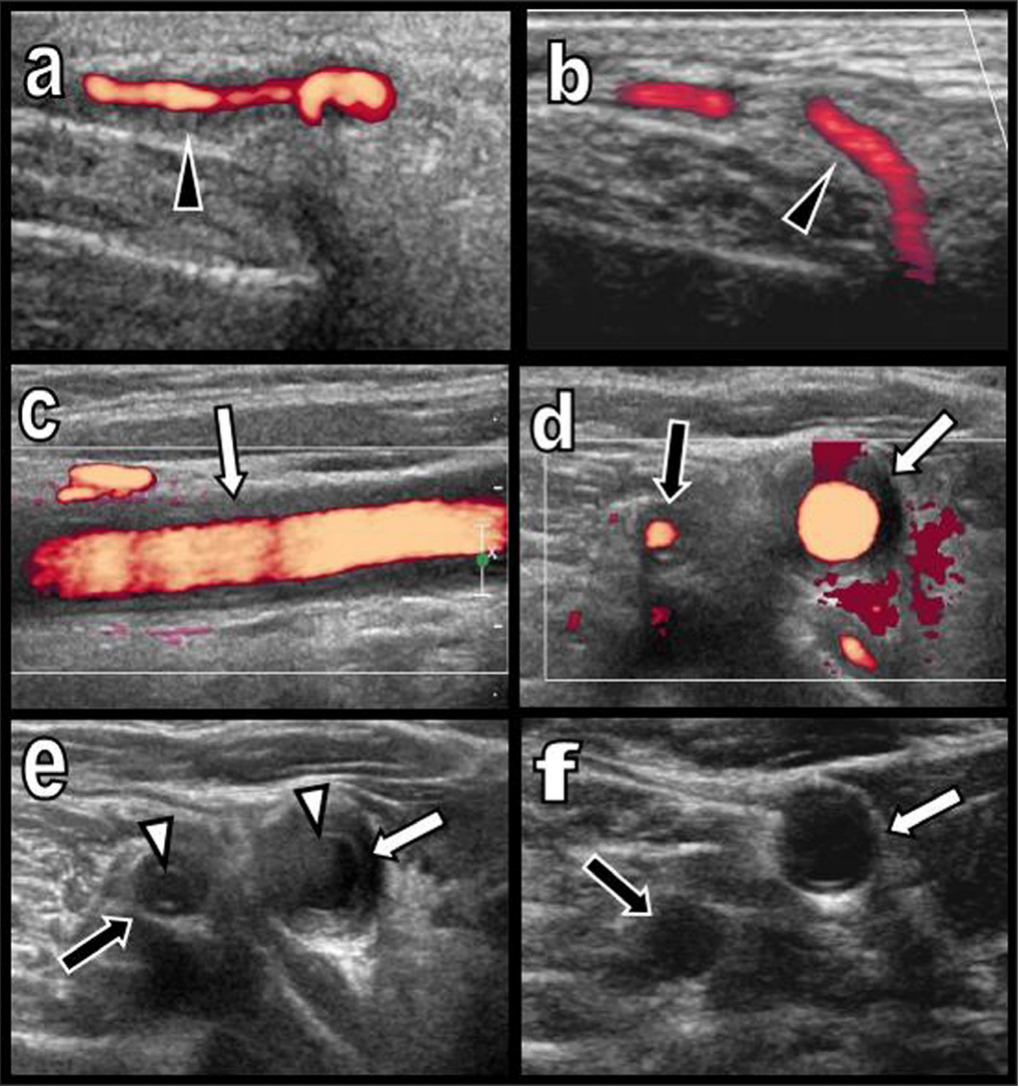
Colour Doppler Ultrasound of the temporal artery before (a) and after treatment (b) and of the right primitive carotid and vertebral arteries before (c, d, e) and after treatment (f).
